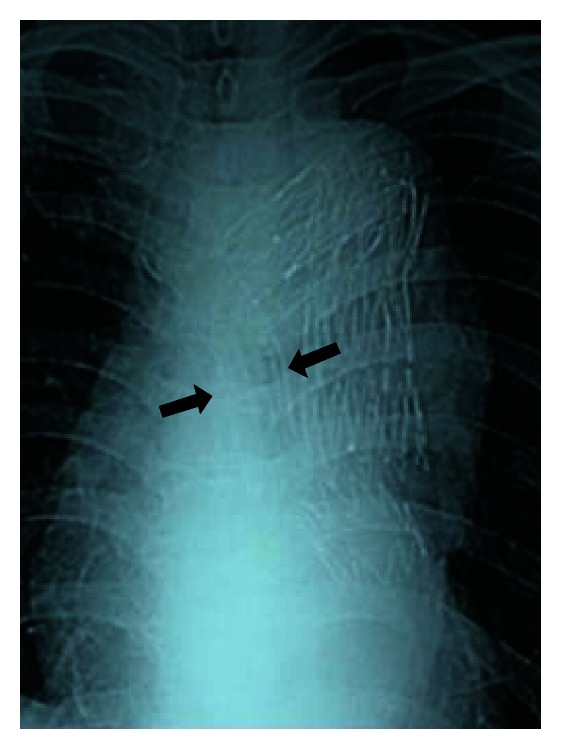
On postadmission day 18, chest X-ray revealed a covered self-expanding esophageal stent (black arrows).
